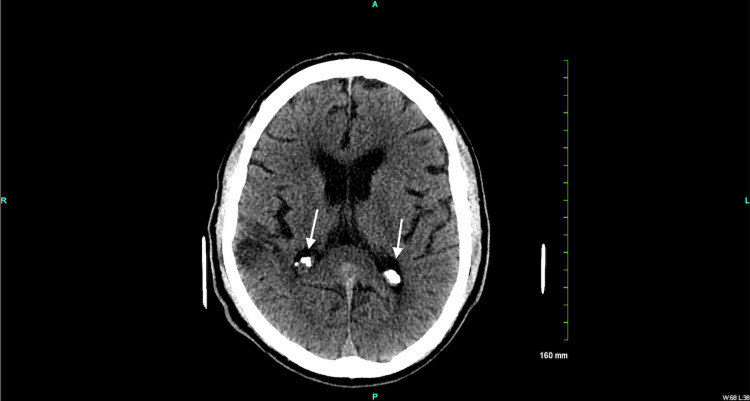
Non-contrast head CT demonstrating intracranial atherosclerotic calcifications (white arrows)
